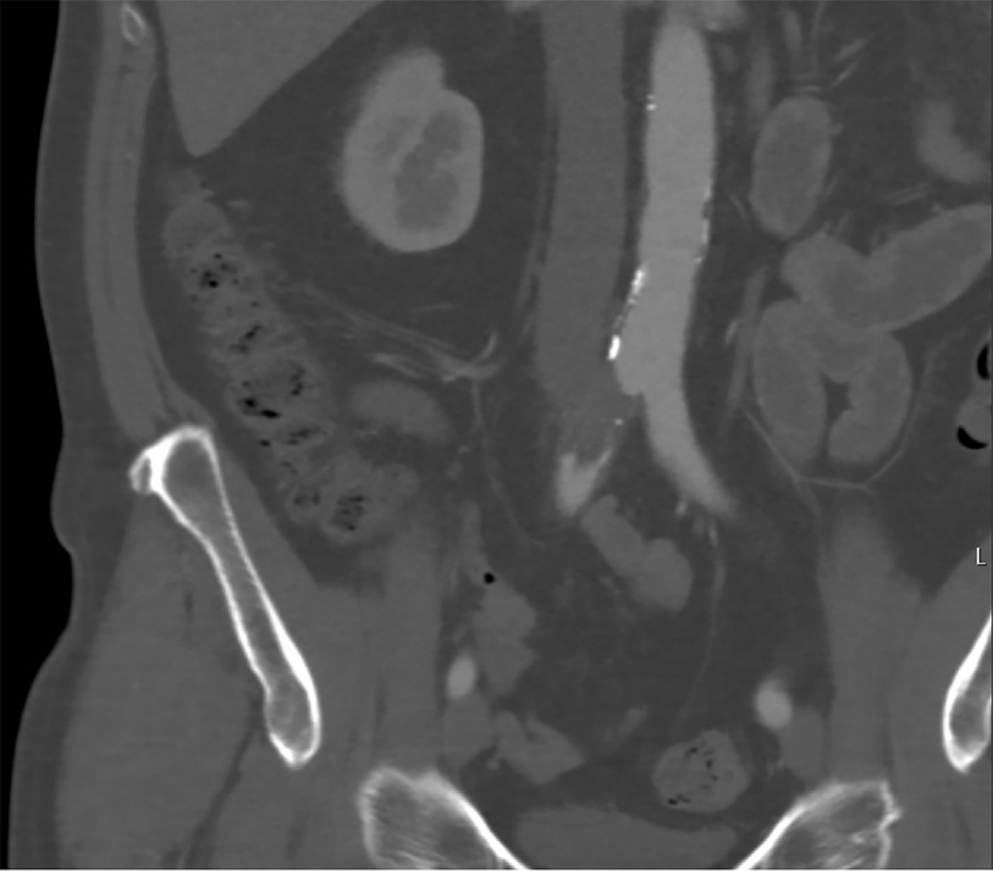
A 50-year-old COVID-19-positive man with no medical history developed acute claudication of the right limb without neurologic deficits 3 days after admission. Computed tomography angiography (CTA) showed short occlusion of the right common iliac artery.
